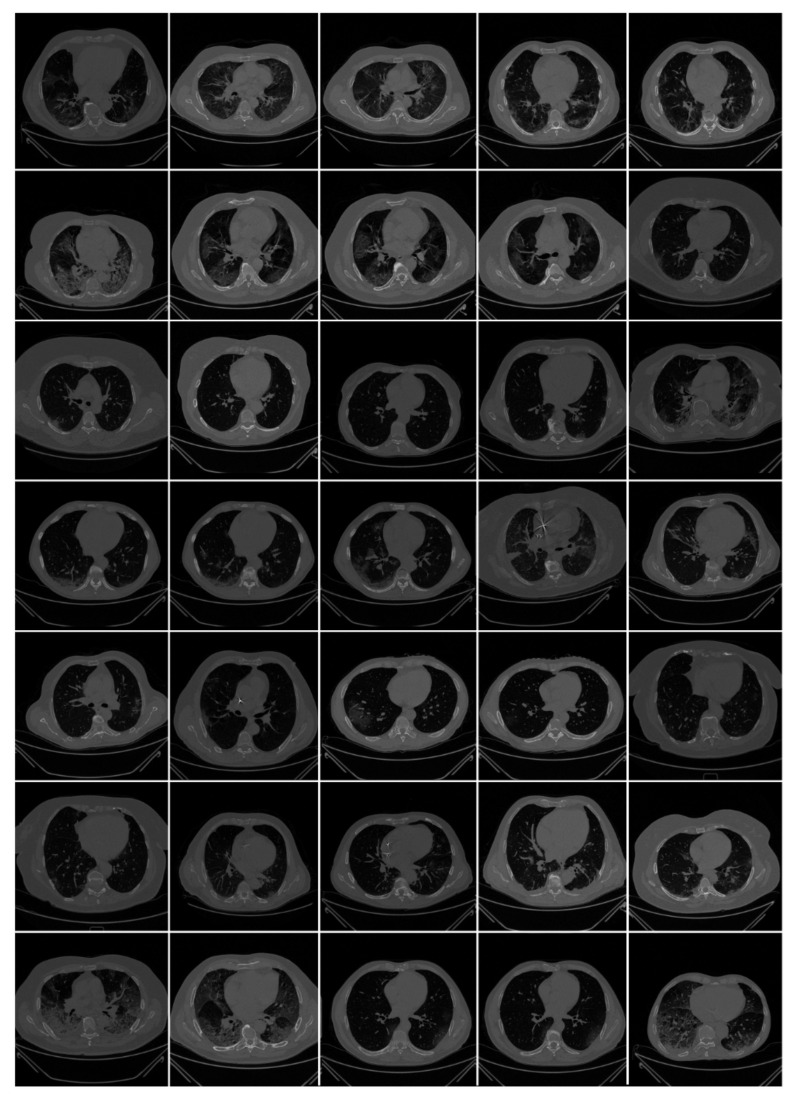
Raw CT images from NOVARA, ITALIAN dataset [3].
